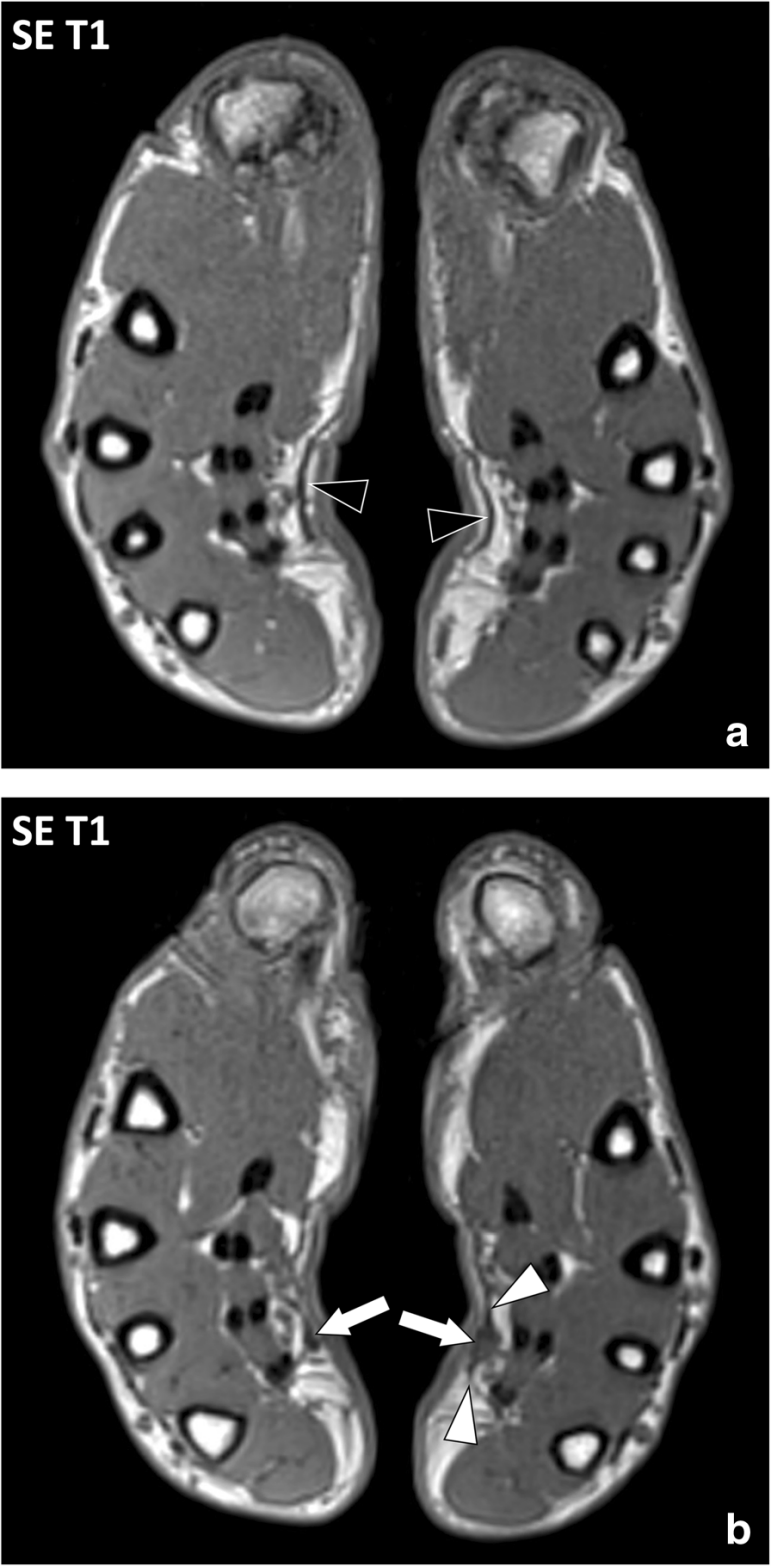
Axial SE T1w images of the hands of a 48-year-old male with palmar fibromatosis. a Palmar aponeuroses are normal in their proximal parts with thin regular margins (black arrowheads). b MRI demonstrates low signal intensity nodules (arrows) in continuity with the palmar aponeuroses (white arrowheads) located in front of the flexor crease of the fourth and fifth fingers corresponding to fibromatosis
